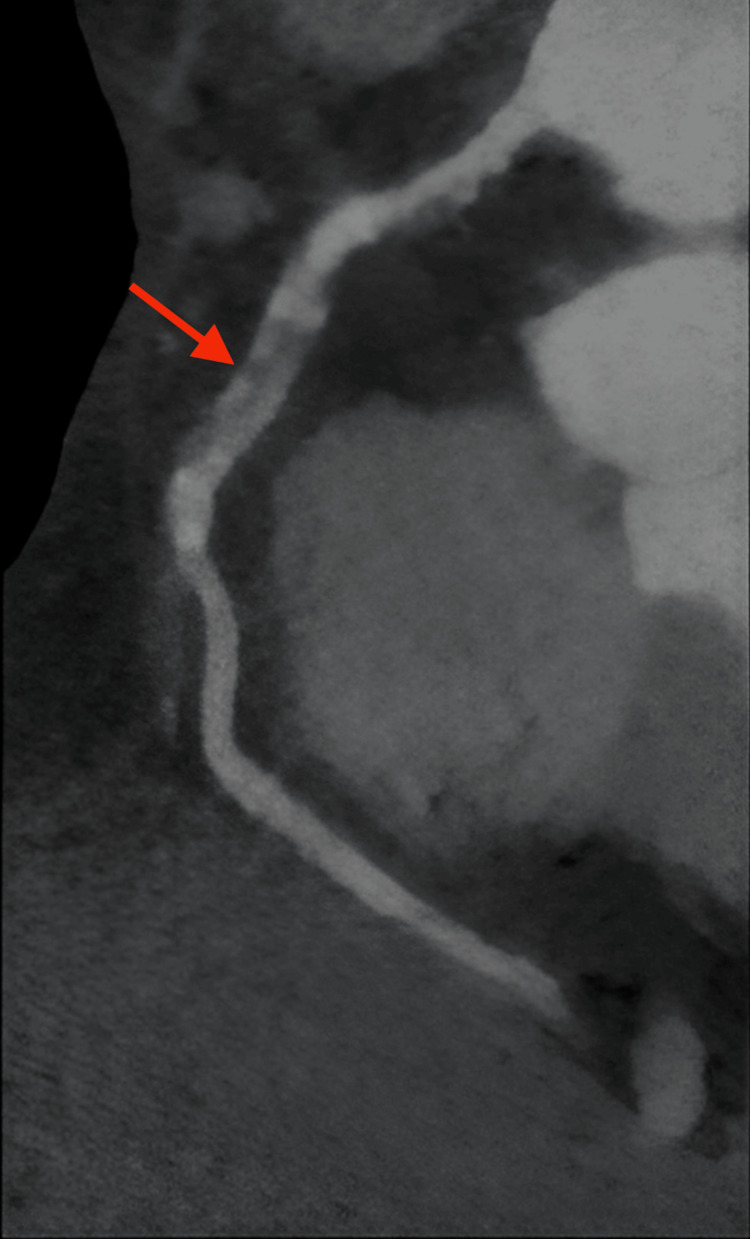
Reconstructed coronary computed tomography angiography image of the right coronary artery. The red arrow indicates a dissected segment of a proximal to middle right coronary artery with partially thrombosed false lumen.
